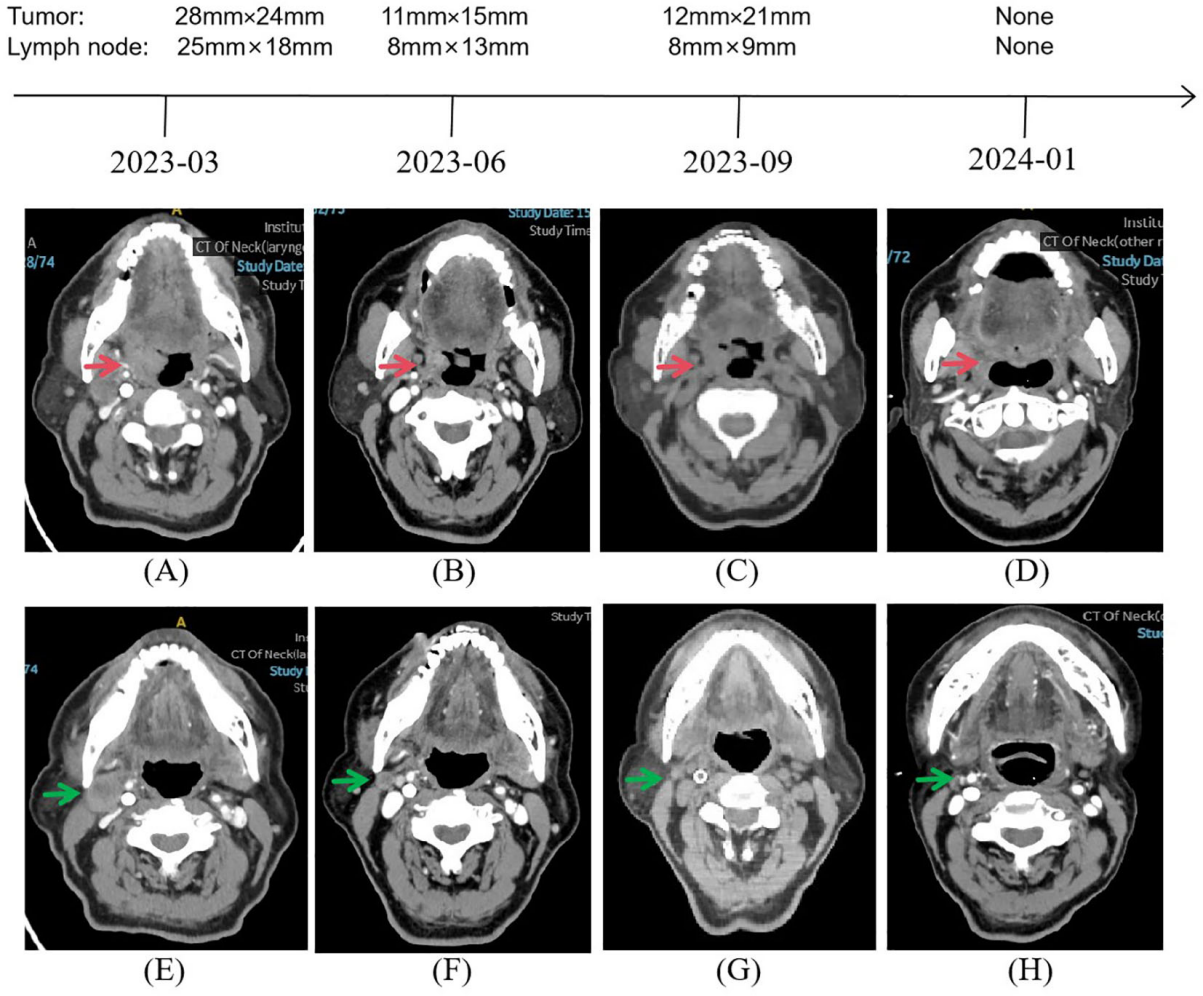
Representative images during the whole treatment. CT imaging exhibited a continued partial response after a total of 8 cycles of camrelizumab plus gemcitabine from March 2023 to September 2023, followed by a complete response under concurrent chemoradiotherapy based on nab-paclitaxel from September 2023 to January 2024. Red arrow showing the tumor. Green arrow showing the neck lymph node.
